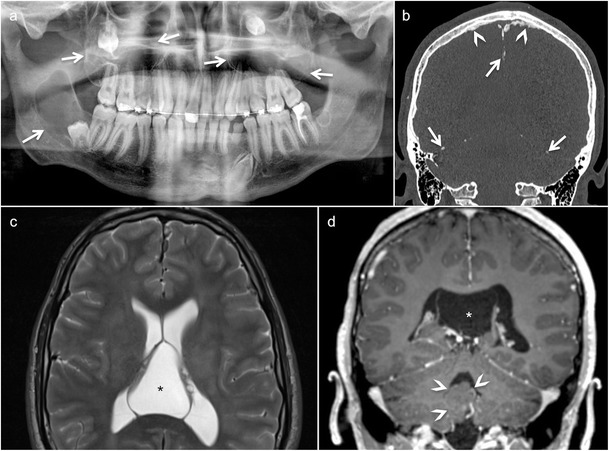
Typical manifestations of nevoid basal cell carcinoma syndrome (NBCCS) in a 16-year-old boy. a Orthopantomography (OPT) shows cystic lesions of the mandible and maxilla (*arrows*), with unilocular and multilocular pattern and smooth or scalloped borders associated with displaced and unerupted permanent teeth. b Coronal CT scan (bone window) shows ectopic calcifications of the falx cerebri and tentorium cerebelli (*arrows*) and spotted meningeal calcifications (*arrowheads*). Brain MRI reveals a cavum veli interpositi on axial T2 (*asterisk* in c) and coronal contrast-enhanced T1 (*asterisk* in d) and also vermian dysgenesis (*arrowheads* in d)
